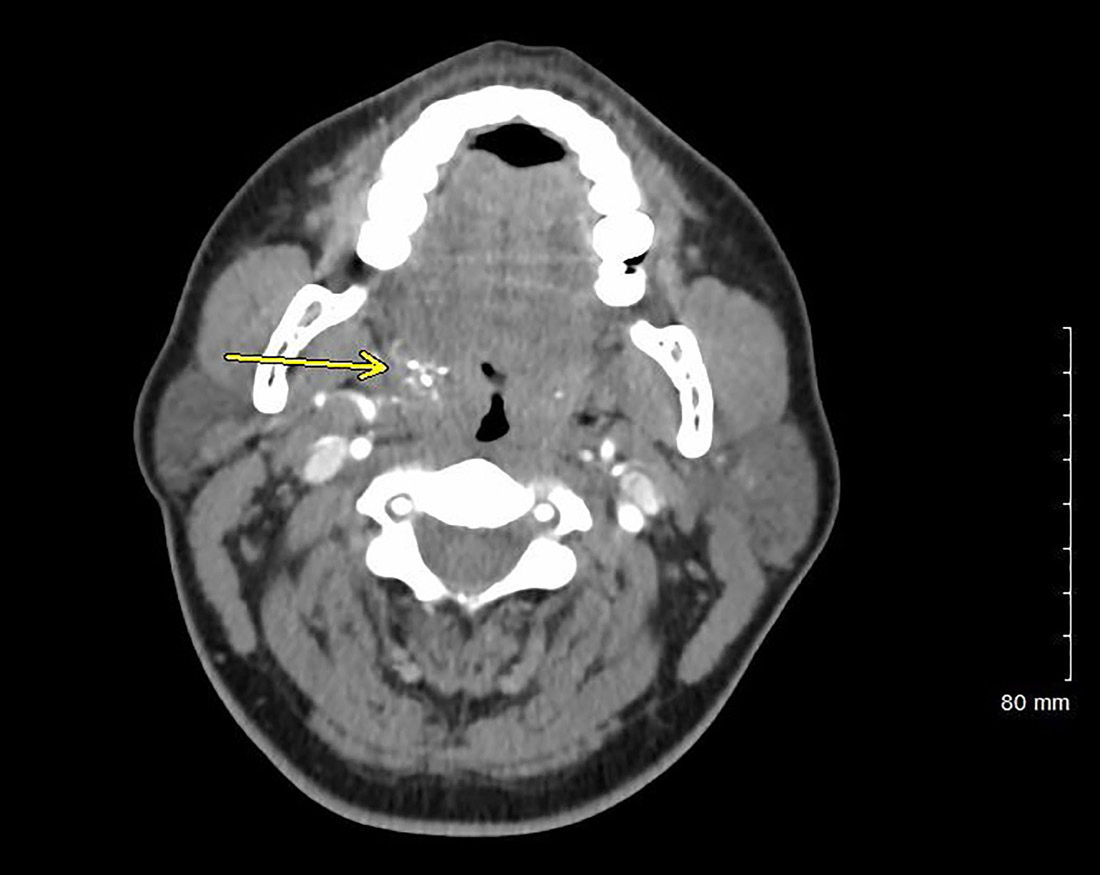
Axial computed tomographic angiography of the head showing palatine tonsillar arteriovenous malformation.
